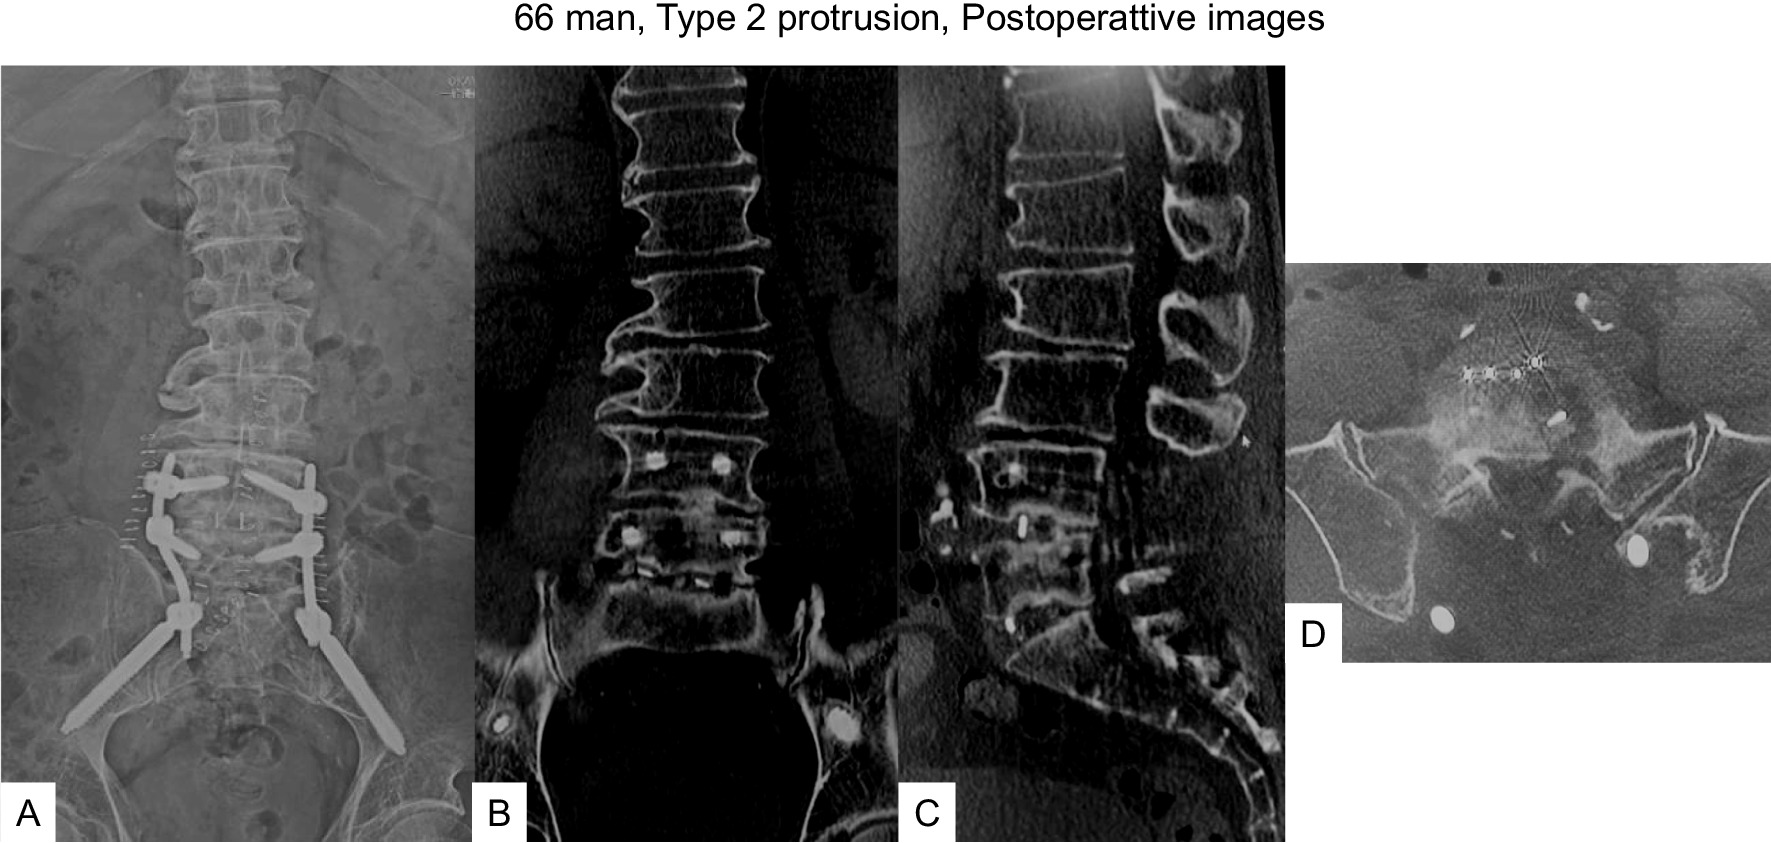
66 man, type 2 protrusion, postoperative images A Final anteroposterior radiogram, B Final coronal reconstruction CT, C Final sagittal reconstruction CT, D Final axial CT at L5/S1
